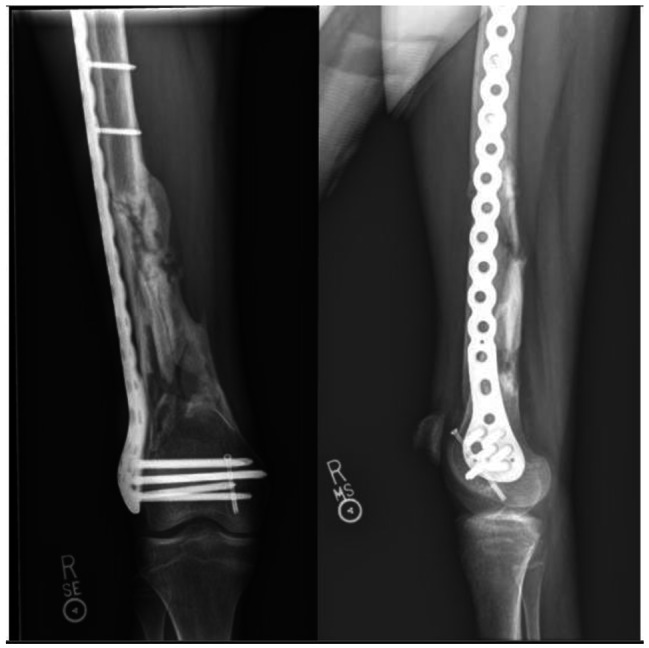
Anterior-to-posterior and lateral x-rays with definitive fixation three months after the same femur fracture demonstrated in Fig. 2. These images show fracture consolidation, healing, and the definitive fixation with plates and screws which were applied two weeks after the external fixator was initially placed at the time of trauma
